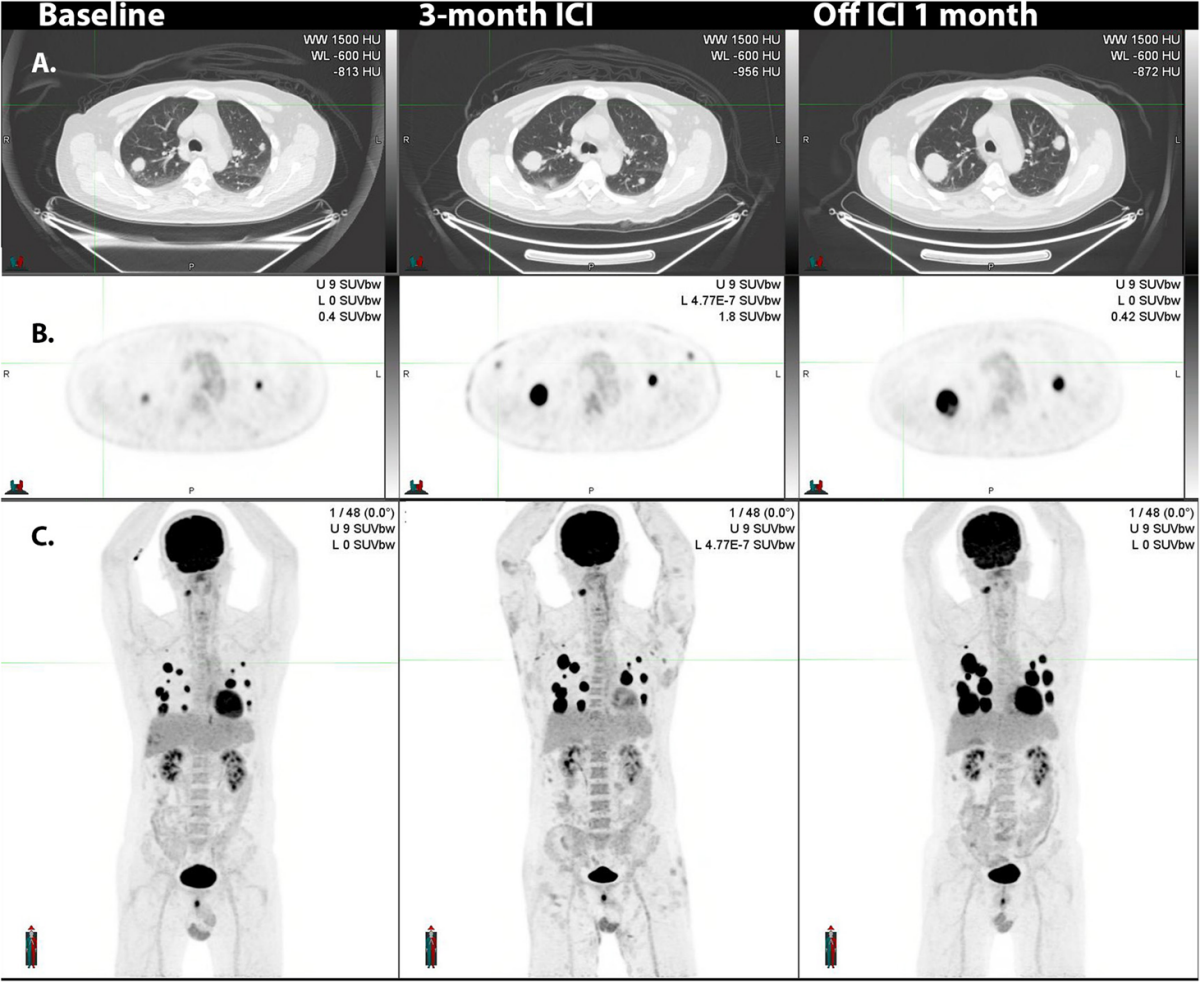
A: in this patient with multiple lung metastases identified at baseline evaluation, which were highly ^18^F-FDG-avid (B). ICI administration led to the development of widespread cutaneous lesions with associated axillary and inguinal nodal uptake apparent on ^18^F-FDG PET/CT. Biopsy confirmed bullous pemphigoid and the ICI therapy was ceased. Serial MIPs demonstrate resolution of the skin changes and nodal changes consistent with an inflammatory basis, but the progression of disease, as suggested already on both CT and PET at early evaluation
